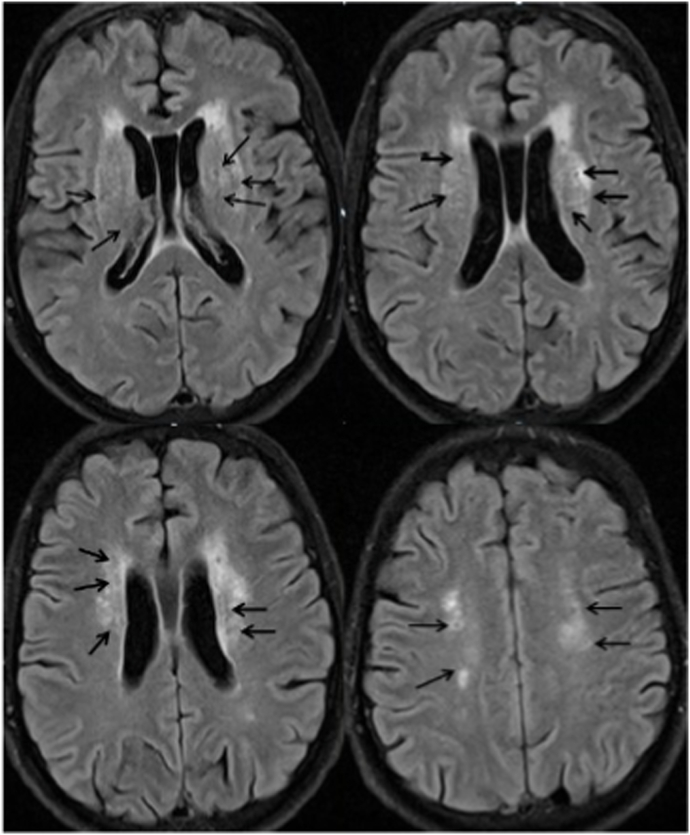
Flair-weighted MRI; White matter hyperintensities around the ventricles with central hypointense in some lesions correspond with periventricular leukomalacia.
